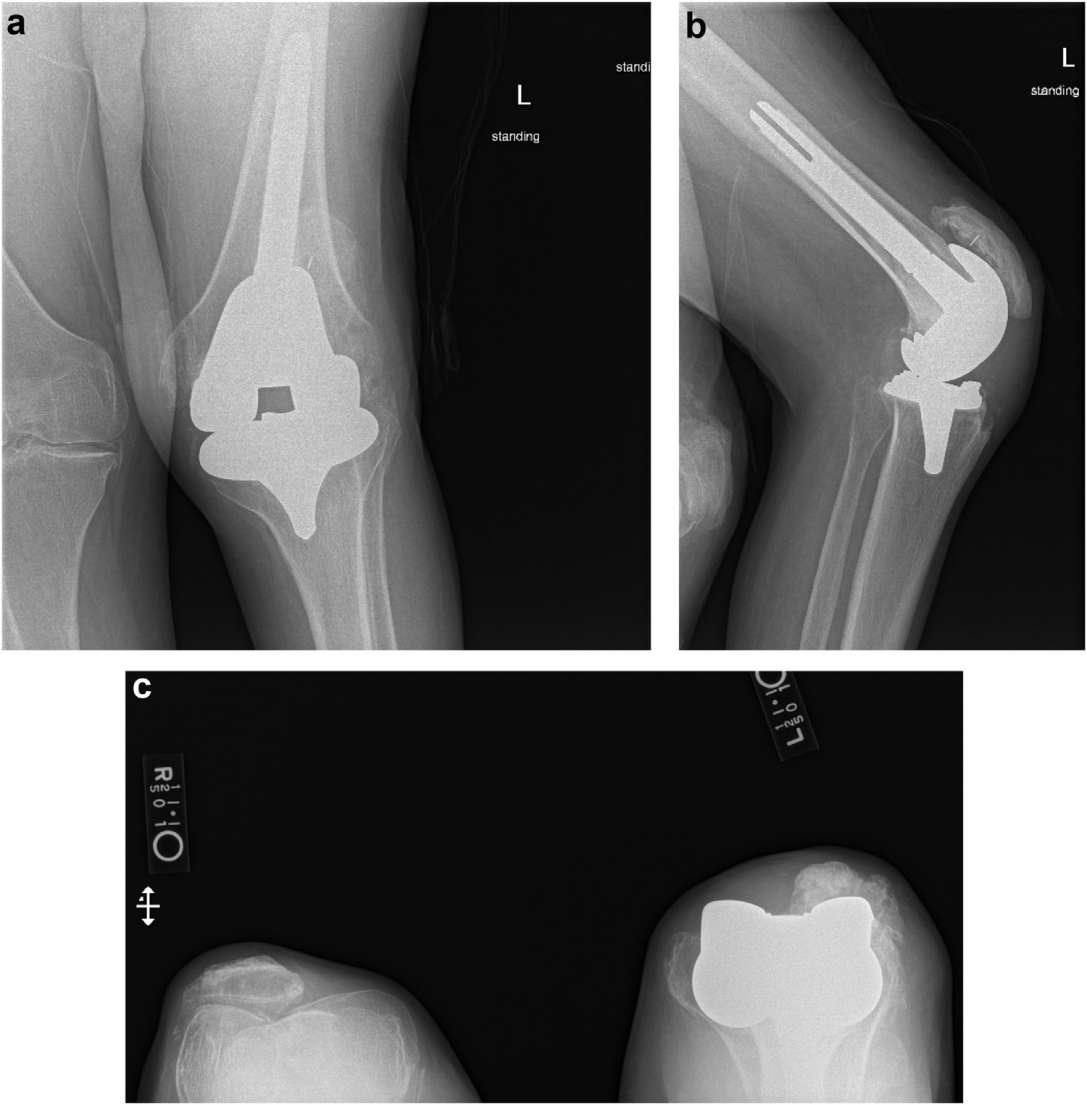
(a) Anteroposterior, (b) lateral, and (c) sunrise postoperative radiographs of left knee 6 months after revision surgery.
